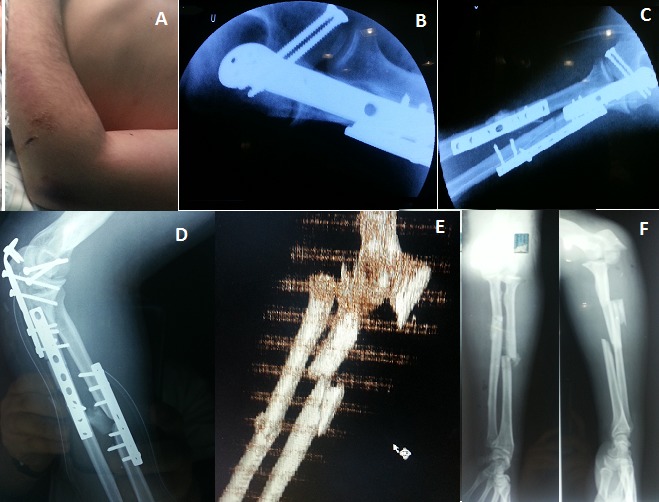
A) image clinique du coude flottant; B) image de scopie per opératoire; C) image de scopie per opératoire; D) radiographie de Contrôle post opératoire; E) TDM avec reconstruction avant bras–coude; F) radiographies standards de l'avant-bras prenant le coude face-profil
